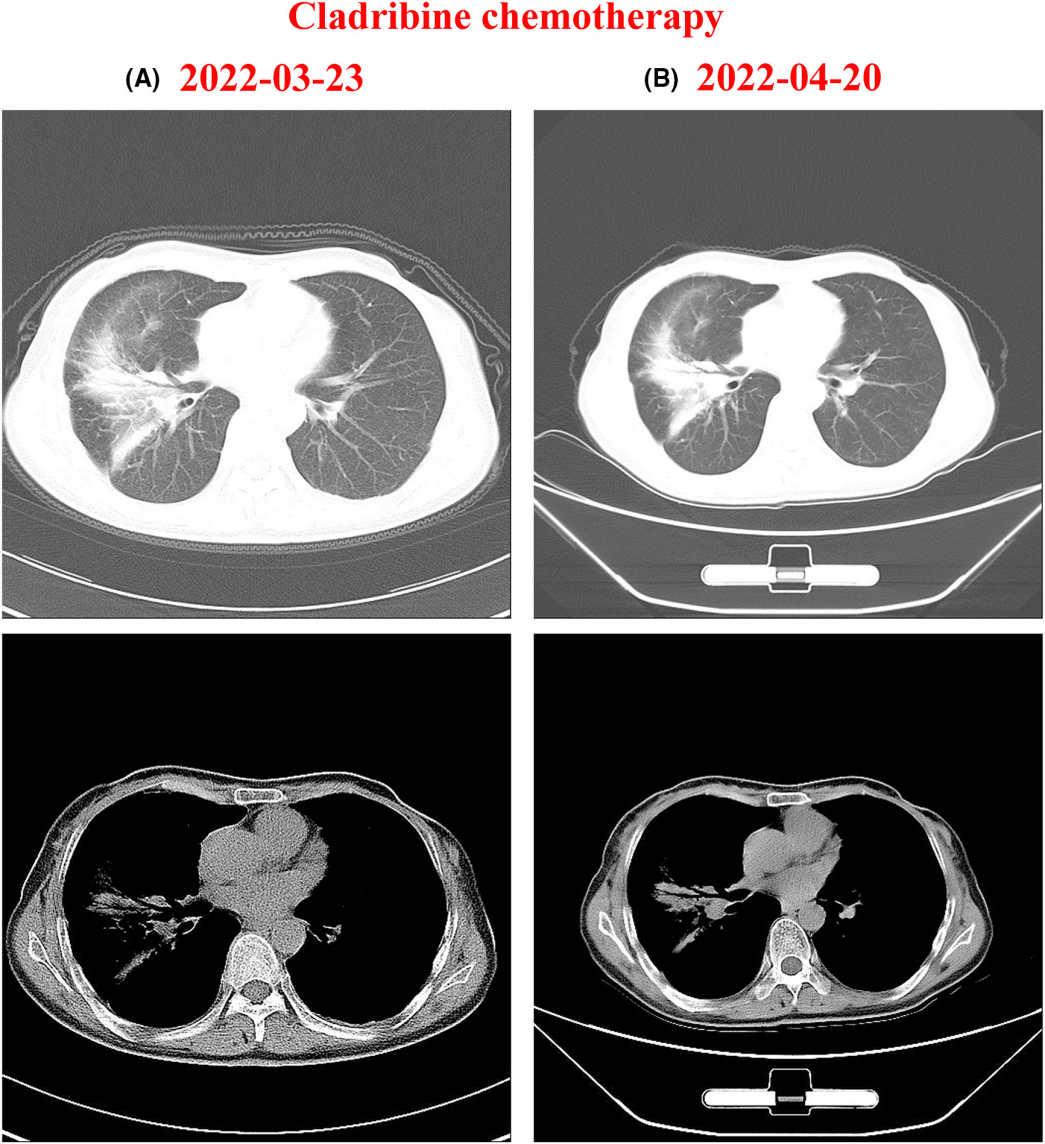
Chest CT scans before and after cladribine chemotherapy. (A) CT scan performed before cladribine treatment. (B) CT scan performed after cladribine treatment (10 mg, Days 1–5).
